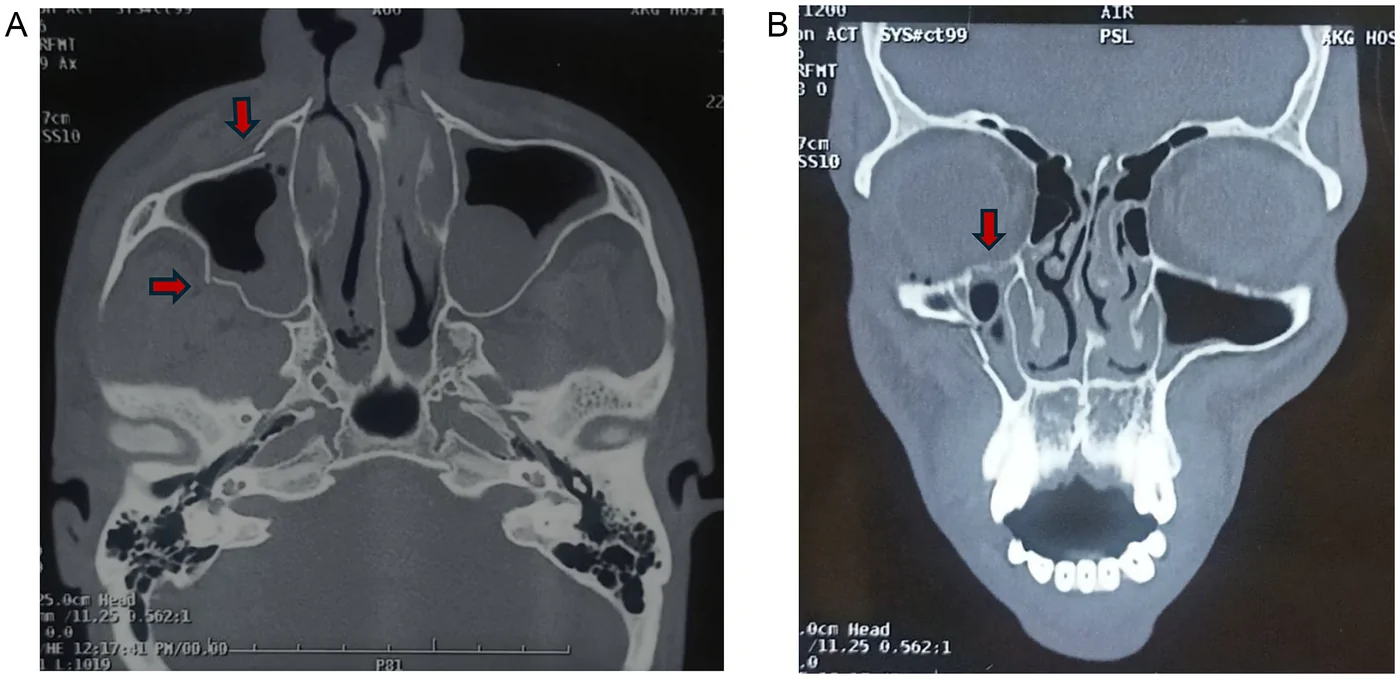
(A) Preoperative CT scan (axial view). (B) Preoperative CT scan (coronal view).
